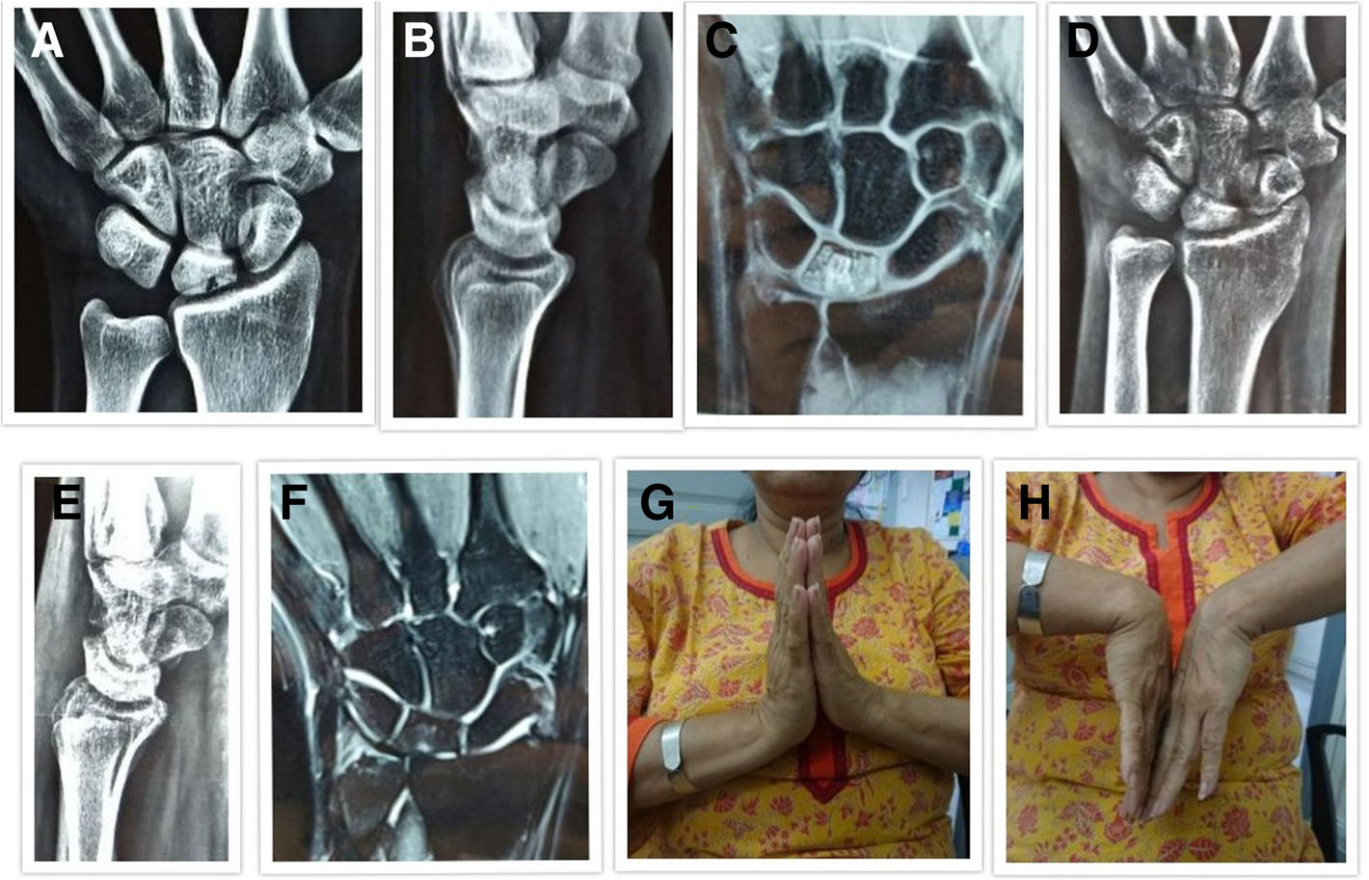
a, b Pre-treatment anteroposterior and lateral radiographs of a 52-year-old female diagnosed with Lichtman stage II avascular necrosis of the lunate left side. c Pre-treatment MRI coronal view of the same patient showing BME. d, e Post-treatment anteroposterior and lateral views at 26 months follow-up showing no radiological collapse. f Post-treatment MRI coronal view showing complete resolution of BME. g, h Clinical images showing good and comparable range of motion of the left wrist joint as compared to normal right side
